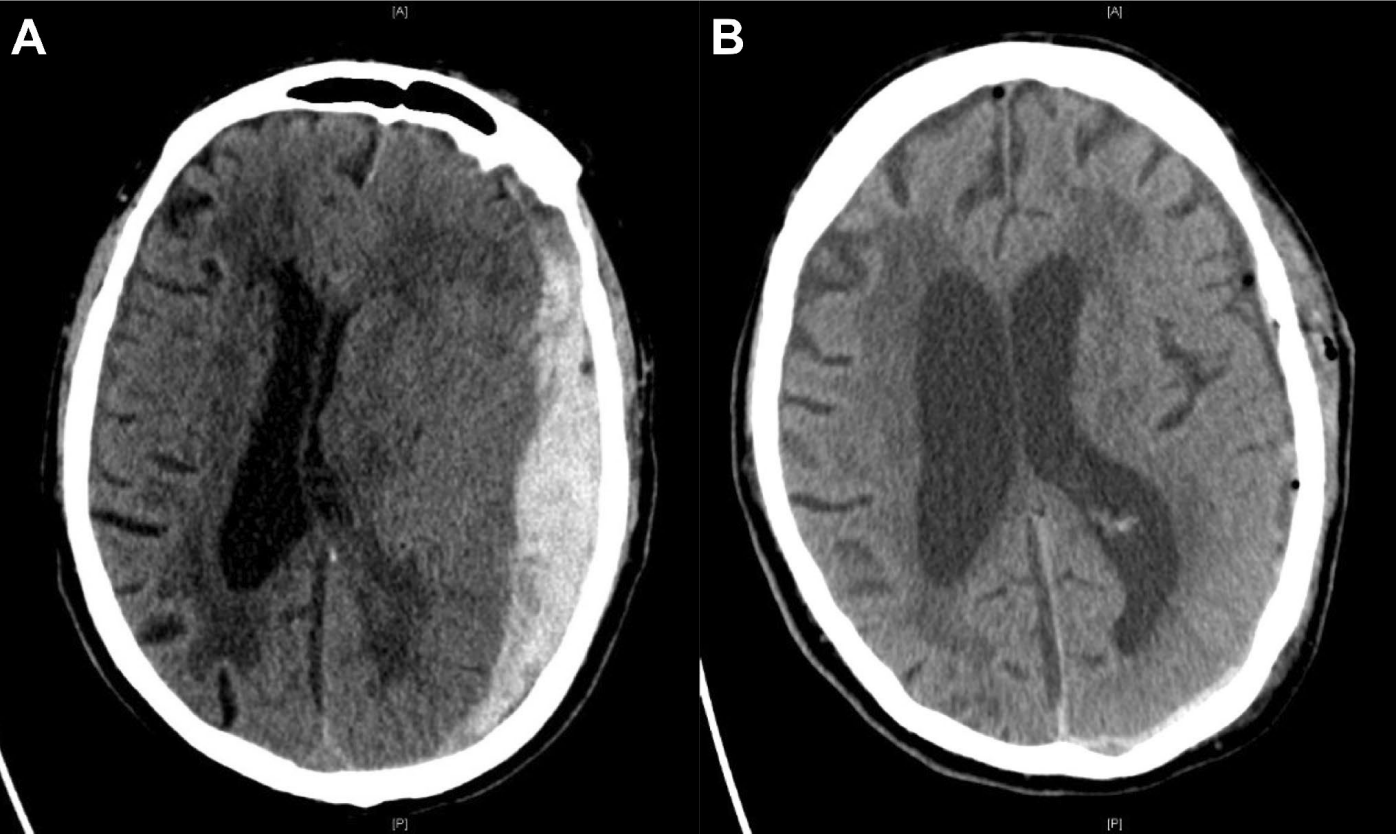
a PreOP computed tomography (CT) of an 80-year-old patient showing a left-sided acute subdural hematoma (aSDH) with a max. diameter of 1.9 cm and approximately 1.3 cm midline shift (aSDH volume = 138 ml). b PostOP CT showing sufficient evacuation of the left-sided aSDH and declining midline shift. GOS score at discharge was 4
